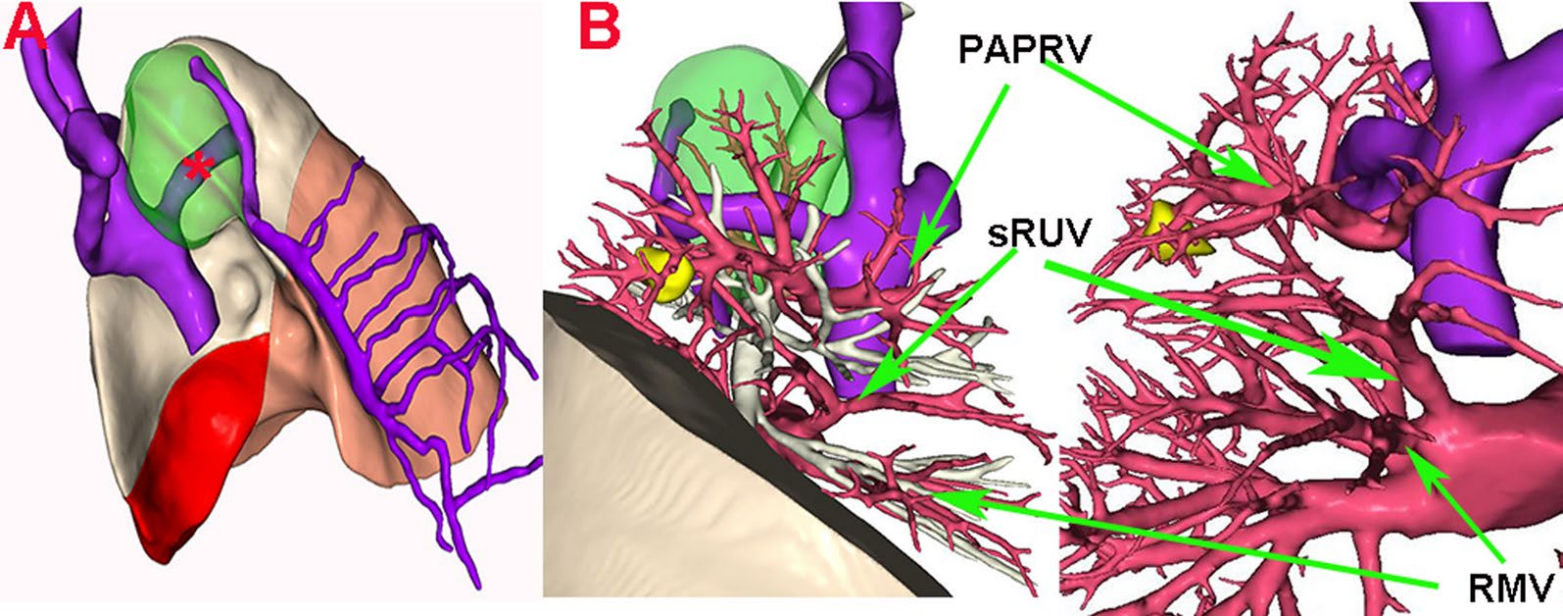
Three-dimensional reconstruction of computed tomography. a Asterisk represents the dilated azygos vein(AV), the green part of the lung is azygos lobe (AL). b PAPVR, partial anomalous pulmonary venous return; sRUV, small branch of right upper pulmonary vein; RMV, right middle pulmonary vein, and the nodule in yellow
